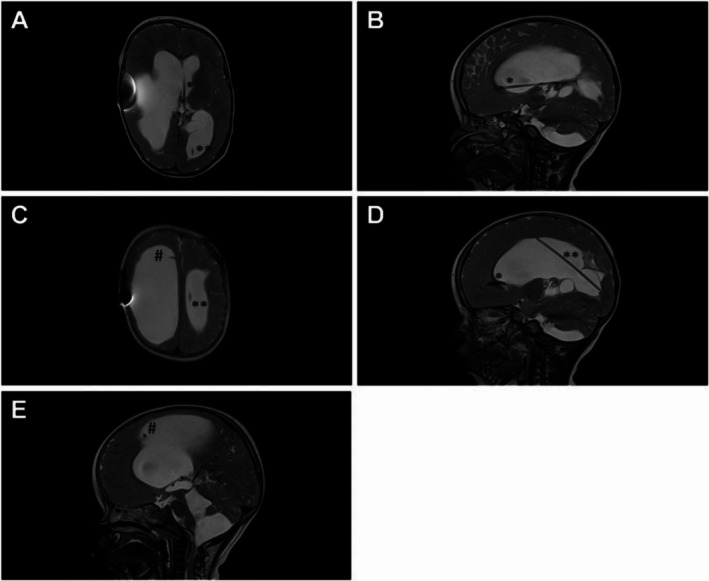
T2 MRI images: A (transversal), B (sagittal) showing frontally the lost catheter on the floor of the left lateral ventricle (*) and in the occipital horn the second lost catheter (); C (transversal) showing the 2nd lost catheter in the left ventricle () as well as on the right frontal side the existing VP shunt catheter (#); additionally, in the sagittal image (D), the lost catheter (*) is shown in the frontal lobe; E (sagittal) enlargement of the 4th ventricle.
